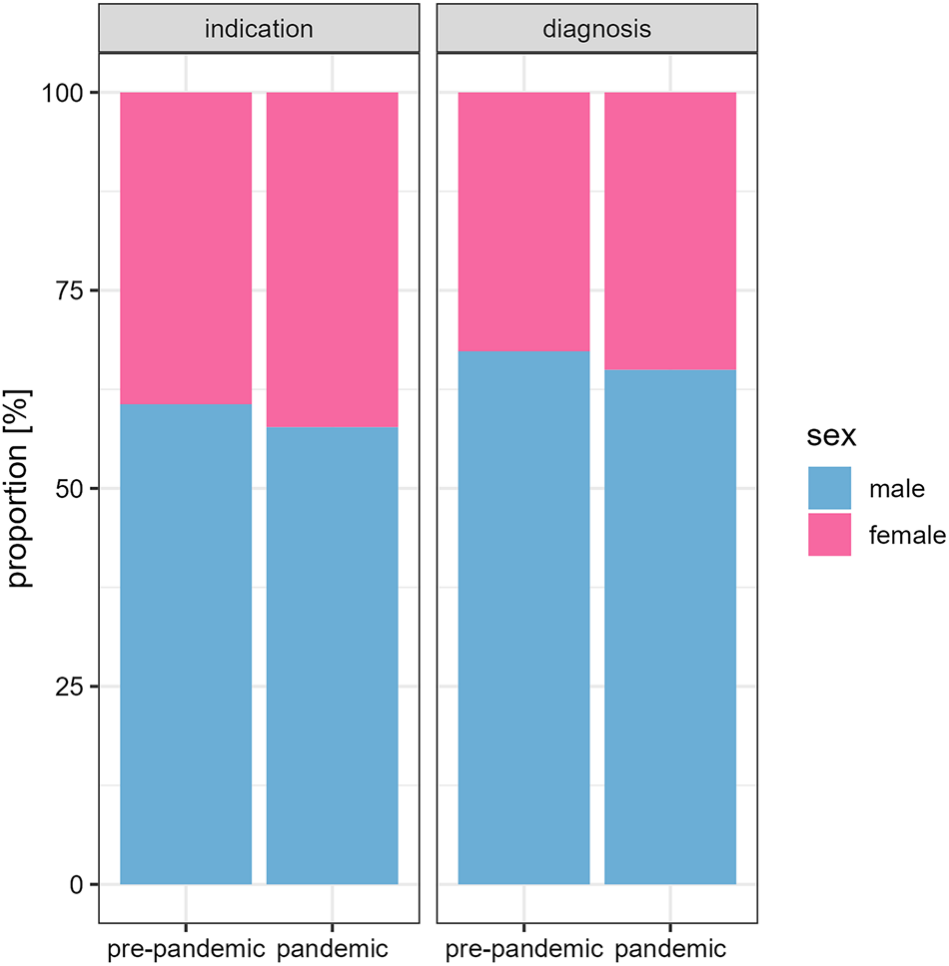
Distribution of sex for the MRI indication of myocarditis and the diagnosis of myocarditis divided in the time before the pandemic on the left and during the pandemic on the right of each pair
